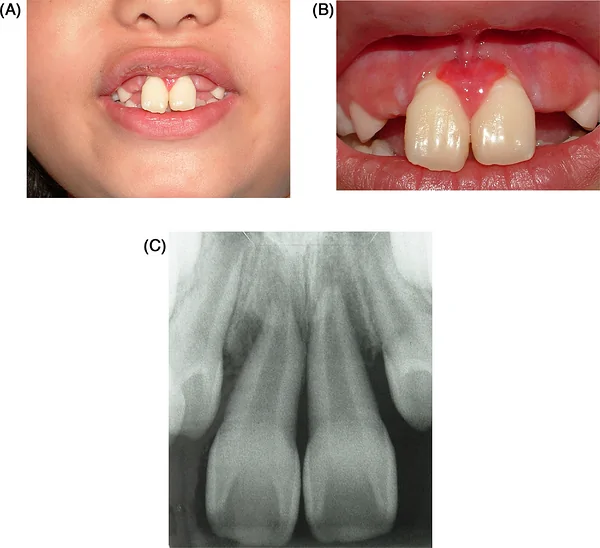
(A) Clinical image from the smile at baseline. (B) Clinical image at baseline. (C) Radiograph image at baseline.
